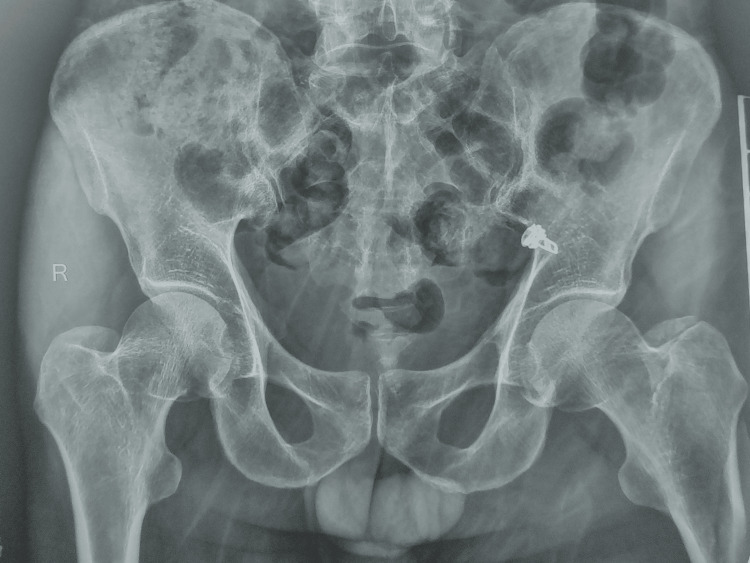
Preoperative X-ray (AP view) showing the pelvis with both hips AP: anteroposterior
